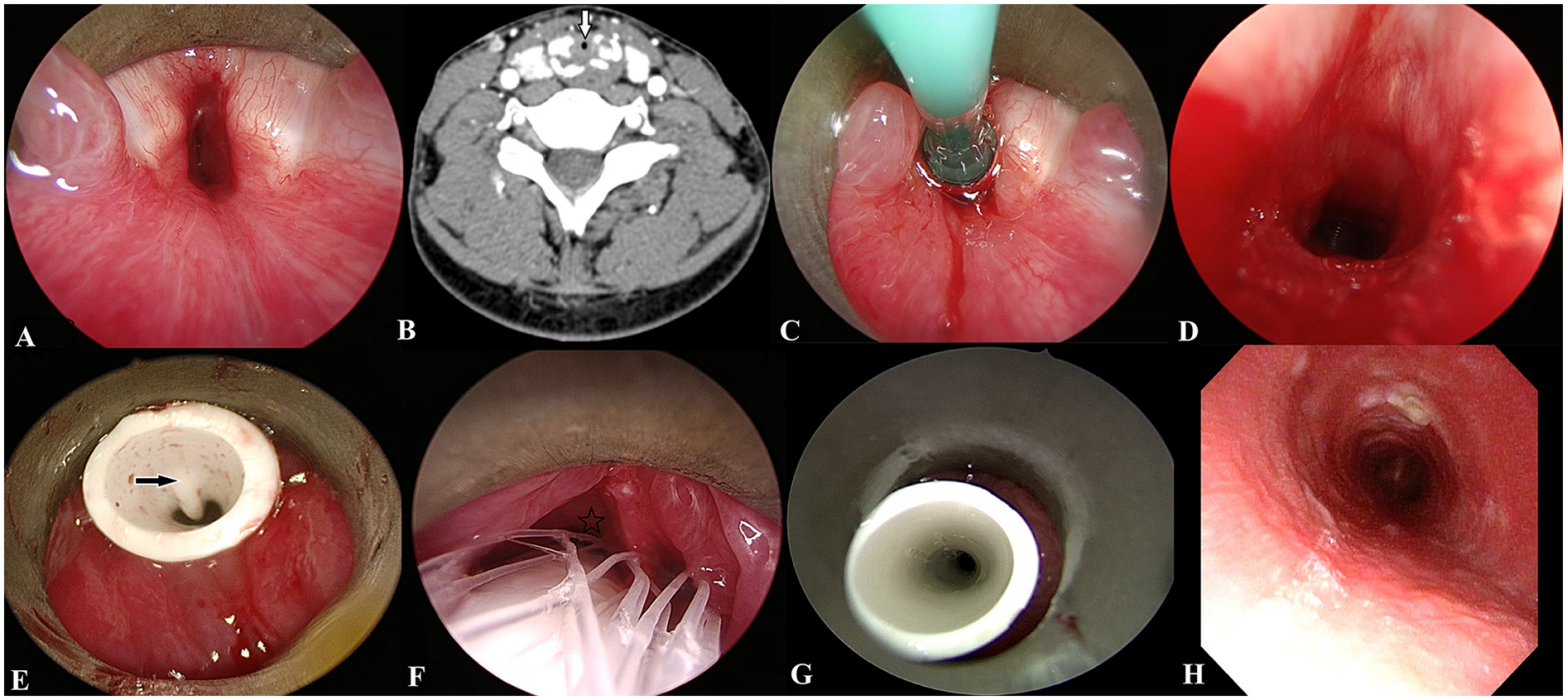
Case 2 before and after treatment. (A) Preoperative endoscopy: subglottic mucosal swelling, airway stenosis. (B) Head and neck CT showed a thickening of the tracheal wall (white arrow) and laryngotracheal cartilage collapse, calcification, and deformity. (C) A balloon of appropriate size was placed at the stenosis for dilation according to the endoscopic exploration under the glottis. (D) Subglottic stenosis improved rapidly after balloon dilation. (E) After T-tube implantation. The black arrow indicates the inner wall of the T-tube fold. (F). Two months after the operation. A drug-loaded stent was implanted between the T-tube and the mucosa of the airway wall, and the asterisk indicates the mucosal gap between the T-tube and the airway wall. (G) Six months after the operation. The inner wall of the T-tube fold disappeared. (H) Subglottic airway was smooth after extubation.
